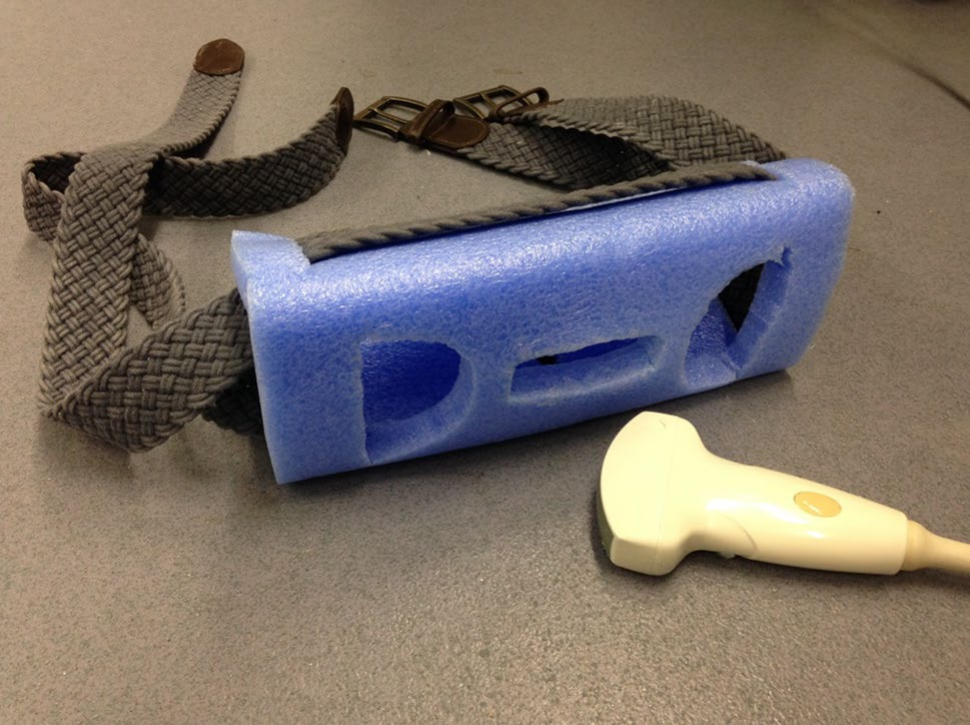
Custom made ultrasound transducer holder and straps
